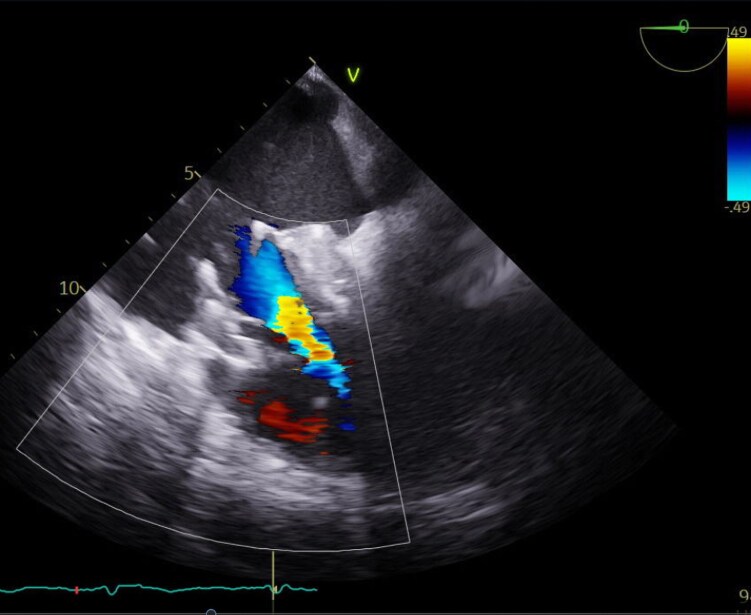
Computed tomography was performed to evaluate sizing parameters (A), as well as to obtain the projected working plane (B). Annular sizing was compatible with a 56 mm Evoque prosthesis. No interaction with the device lead was expected.
